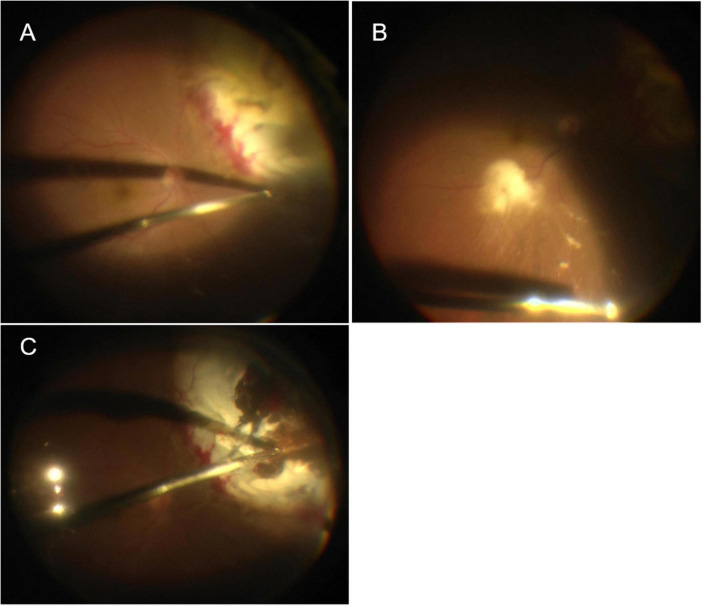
(A) The orbital CT showed a foreign body, about 20 × 12 mm in size, partially involving the nasal ocular wall; (B,C) the foreign body was removed directly from the orbit outside ocular and the ocular wall was intact after removal.
